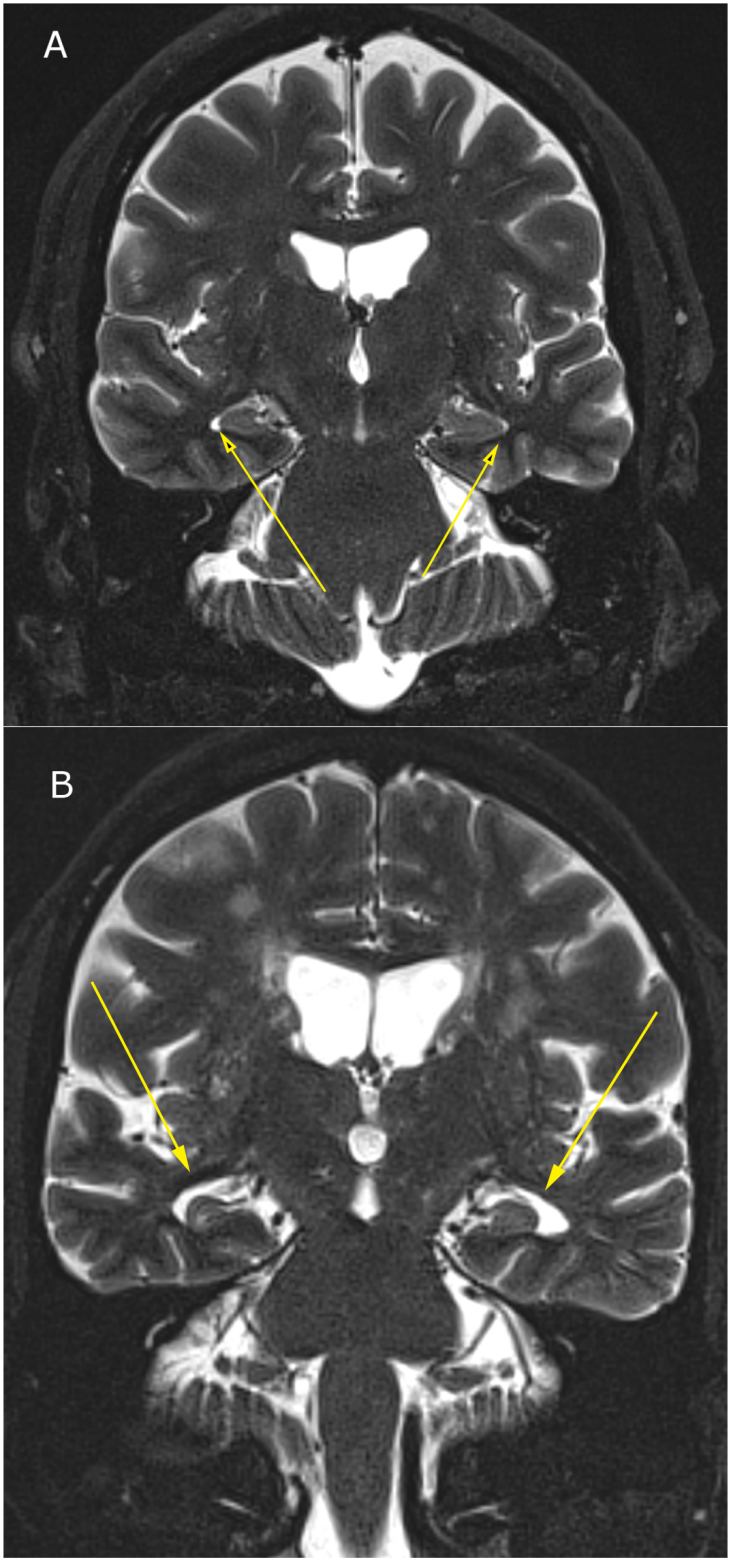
3T MRI of inferior lateral ventricular enlargement due to temporal lobe atrophy; (2a) Normal inferior lateral ventricular size in 55-year-old; (2b) Severely enlarged inferior lateral ventricles due to temporal lobe atrophy in 68-year-old male.
